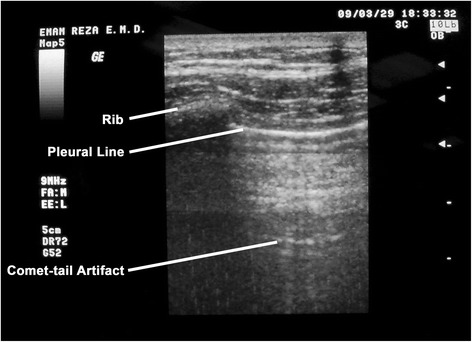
Ultrasound pattern of the normal lung in B-mode with a linear transducer. The pleural line is depicted as the hyper echoic line limited between two sequential ribs. The backward and forward movement of pleural layers against each other occurs on the same level as the pleural results in lung sliding. The comet-tail artifact is a reverberation artifact arising from the pleural line and appears as a hyper echoic line parallel to the pleural line and at the depth of the parenchyma
